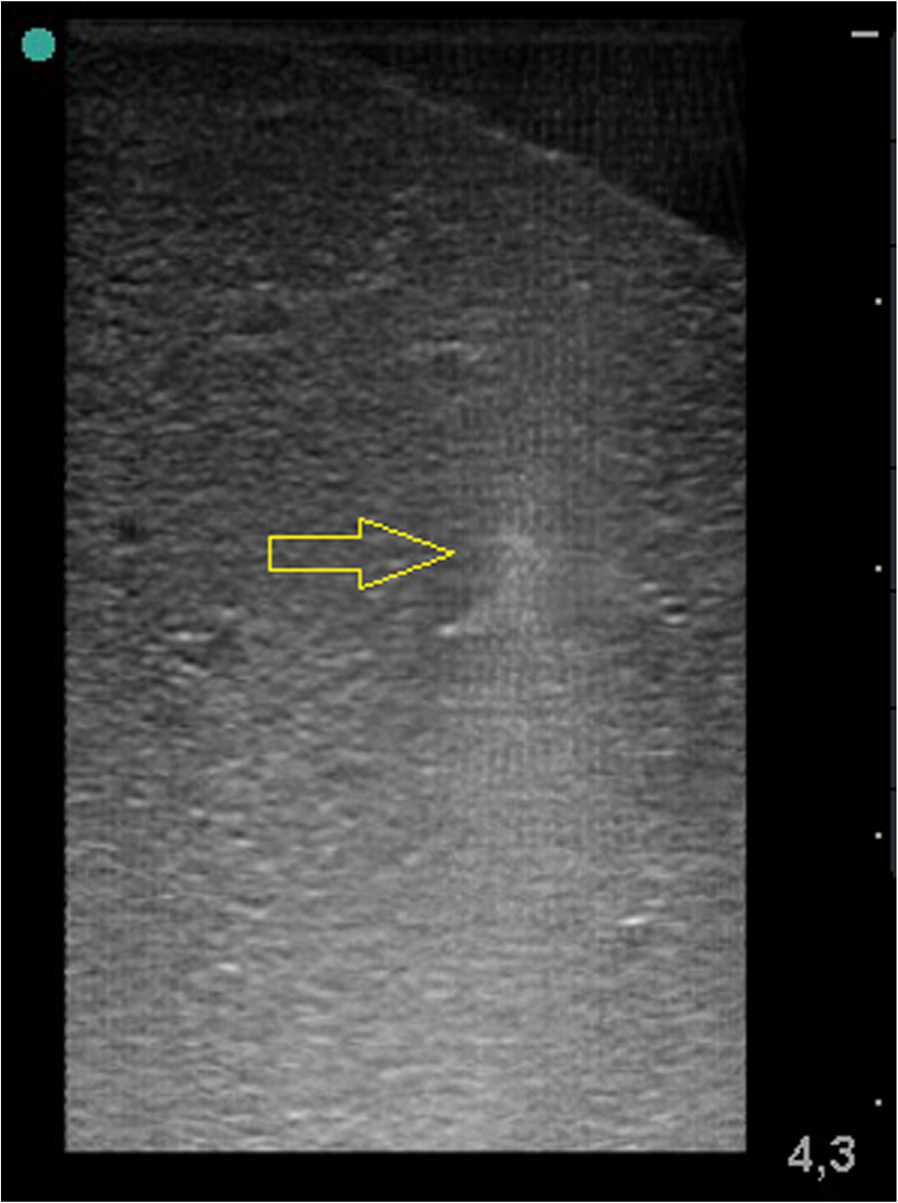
Sonographic manifestation of a cavitation cloud in the focal zone; *arrows* indicate the focal position
